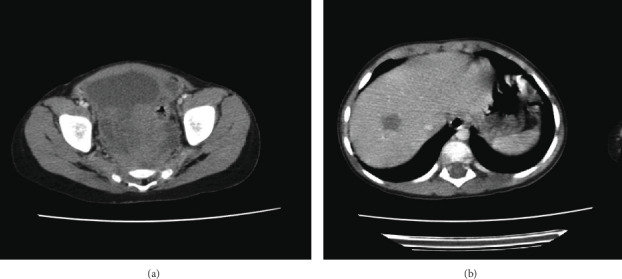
CT scans showing the tumor (a) and the metastatic liver lesion (b) at diagnosis.
